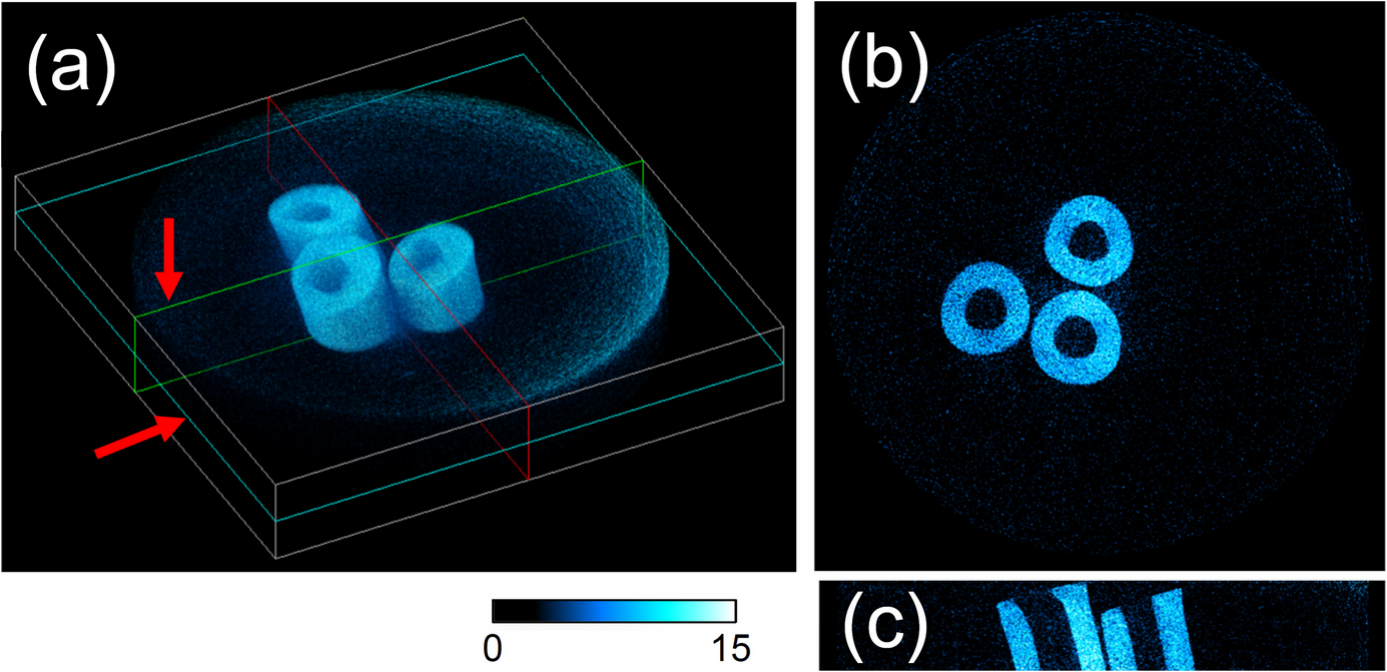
Computed tomography of lead-free solder with a data acquisition time of 2.45 ms. (*a*) Three-dimensional reconstruction. (*b*, *c*) Cross-sectional views of the light blue and green sections as indicated by the red arrows in (*a*). Mechanically cut edges are visible at the top of the reconstructed volume. The dimensions of the white box in (*a*) are 4.1 mm × 4.1 mm × 0.67 mm. The color bar represents the linear attenuation coefficient (cm^−1^) for (*b*) and (*c*).
